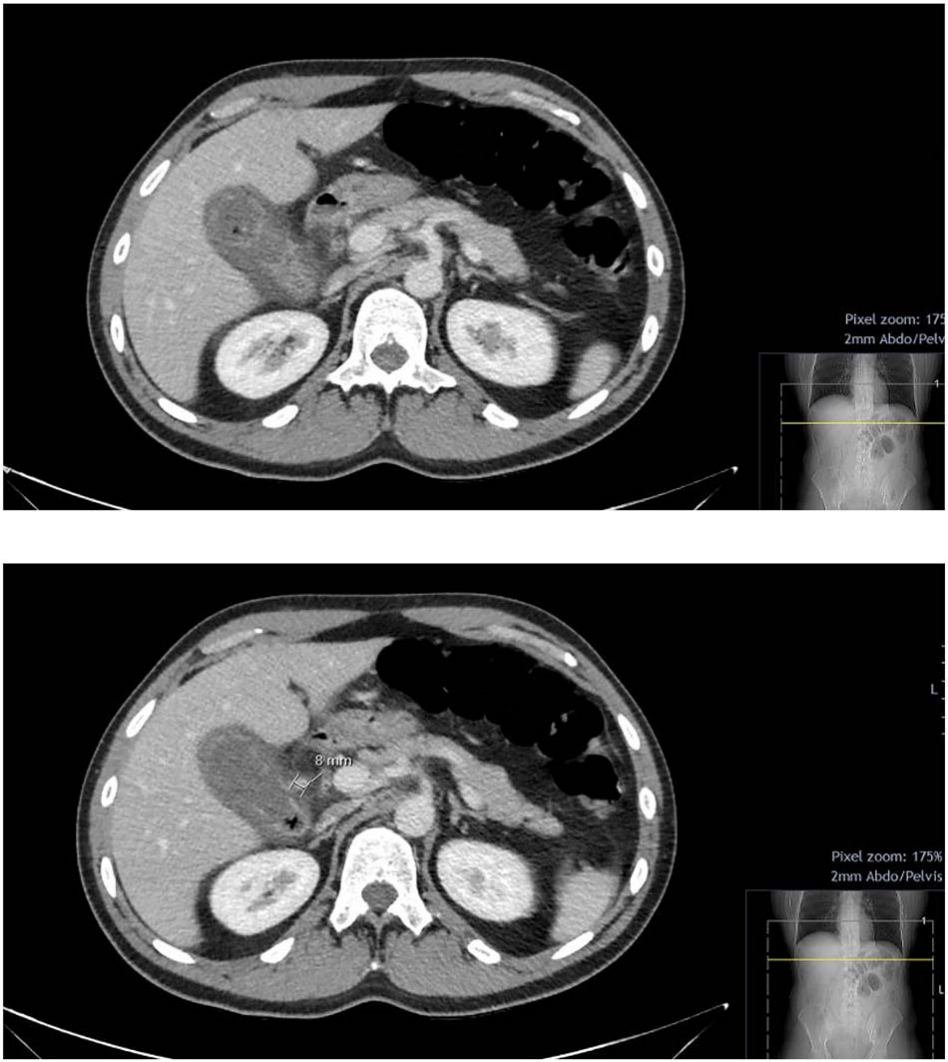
CT abdomen and pelvis with contrast; hyper-enhancing, oedematous gallbladder wall with fat stranding and gas locules consistent with acute calculous cholecystitis; suspected pseudoaneurysm of the cystic artery measuring 7.6 mm.
